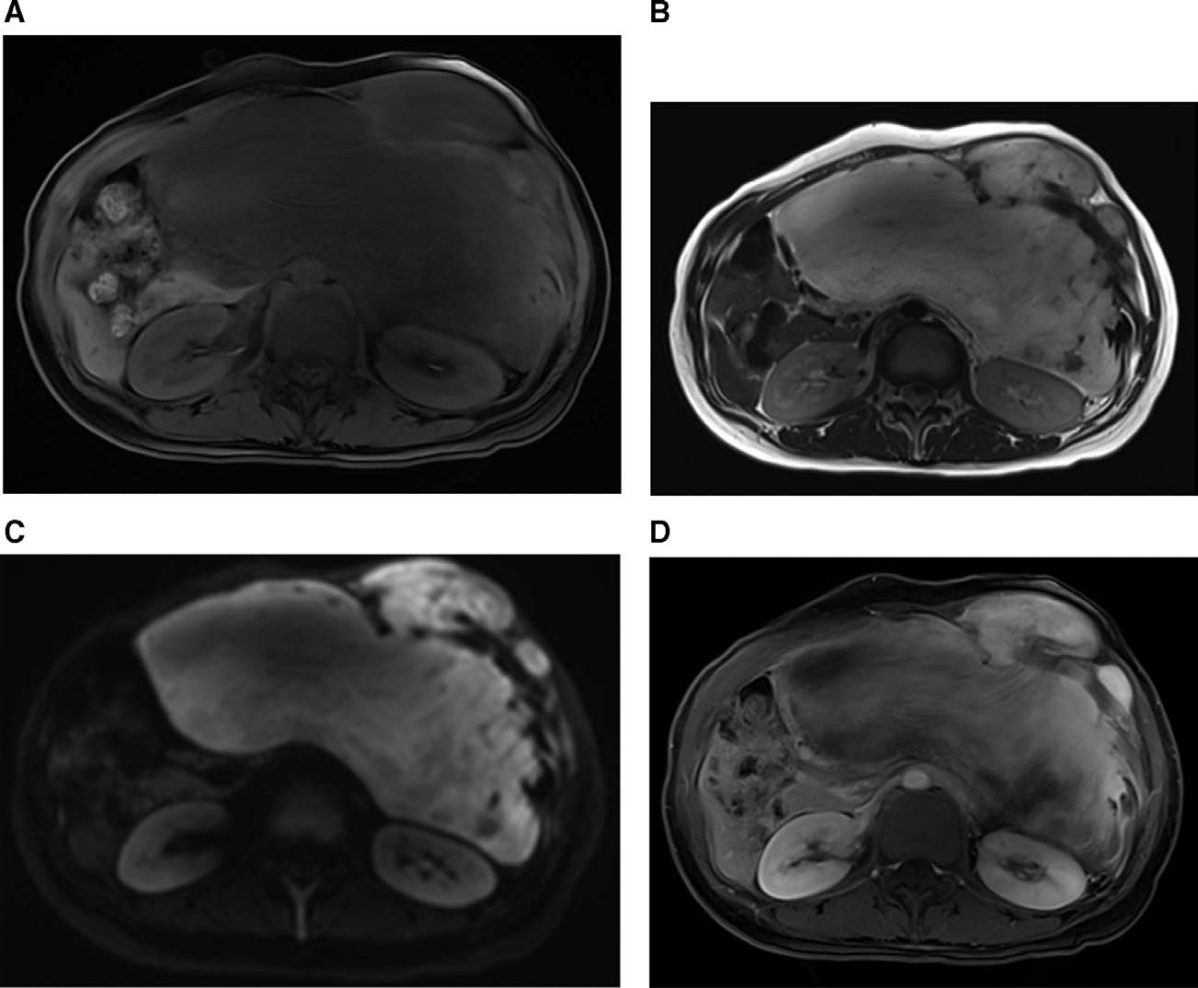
Diffusion-weighted and contrast-enhanced abdominal magnetic resonance imaging. (A) T1 weighted MRI image. The mass showed that low signal intensity with abdominal wall and left 8th to 10th ribs invasion. (B) T2 weighted MRI image. The mass showed that bright signal intensity and heterogeneous component. (C) Diffusion-weighted MRI image. The mass on abdominal wall showed focal bright signal intensity, but heterogenous and low signal intensity was noted on intra-abdominal component of mass. (D) T1 weighted MRI image on 3 minutes delayed phase. Bright but heterogenous signal intensity with gradual and slow enhancement was noted.
